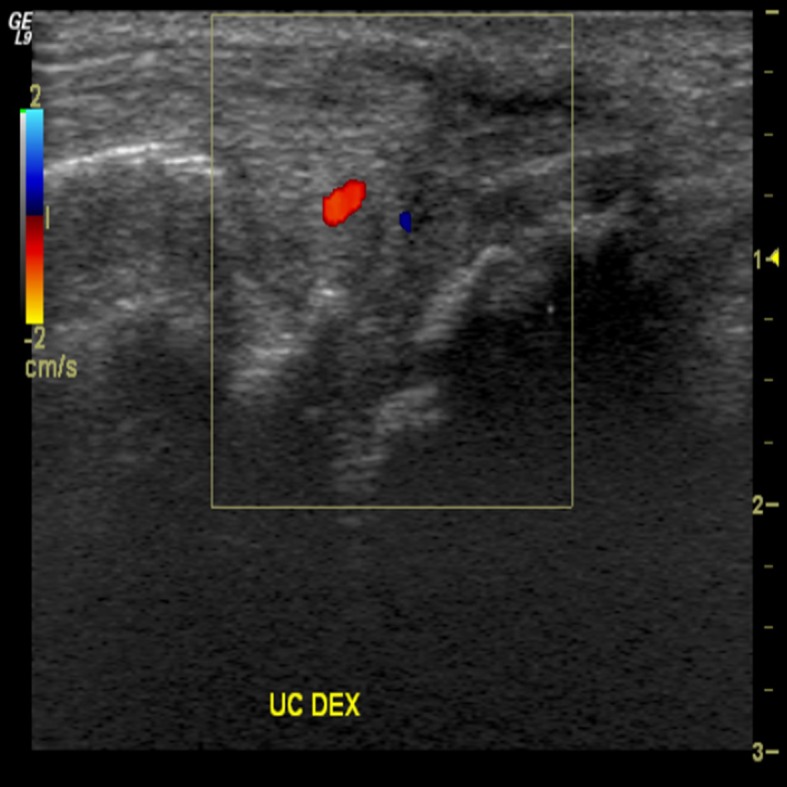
Vascularization in ulnocarpal joint – group 1: Doppler color signal in only one blood vessel.
